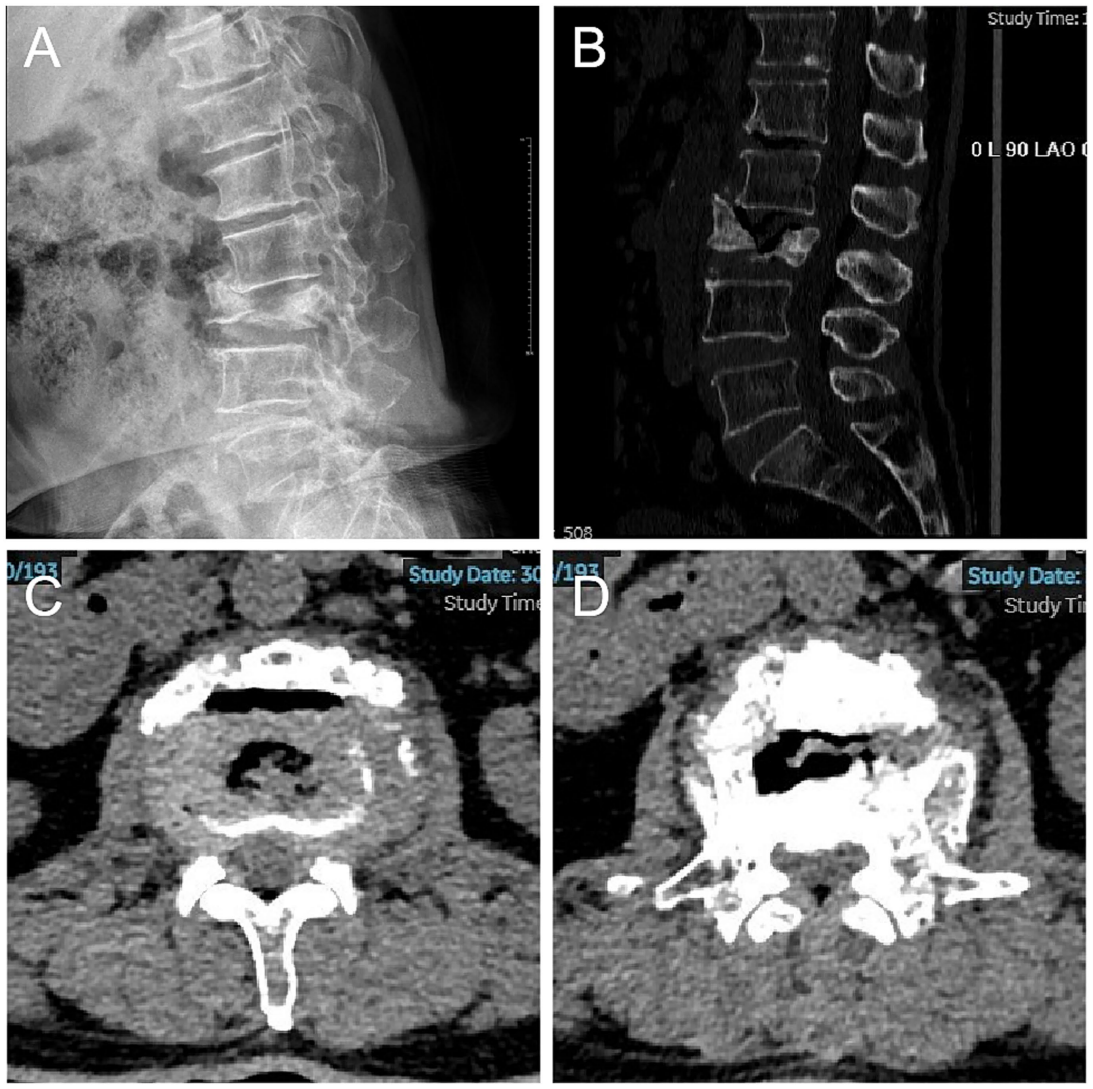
Preoperative radiographs. (A) The preoperative lateral lumbar spine radiographs (DR) revealed an L3 vertebral compression fracture with collapse of the L2/3 intervertebral disk space and a focal kyphotic deformity. (B–D) Preoperative CT of the lumbar spine further confirmed the L3 vertebral body fracture, accompanied by spinal canal stenosis at the L2/3 level.
